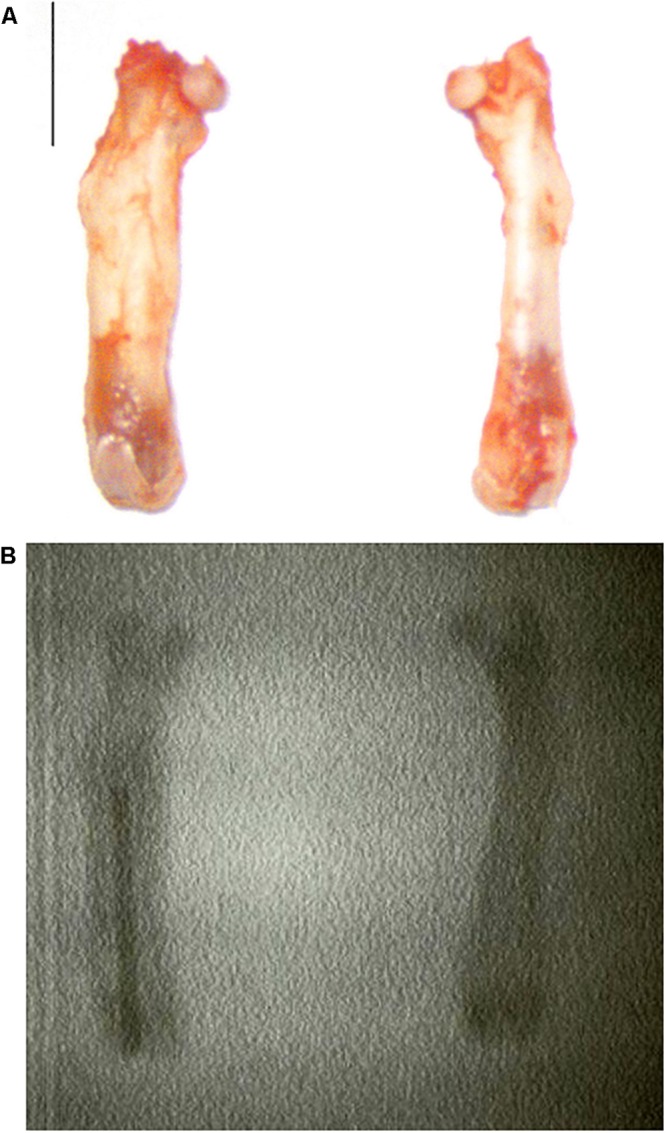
Photograph of a femur without (A, right) and with osteomyelitis (A, left) and their radiographic image (B). The bar represents 0.5 cm.
